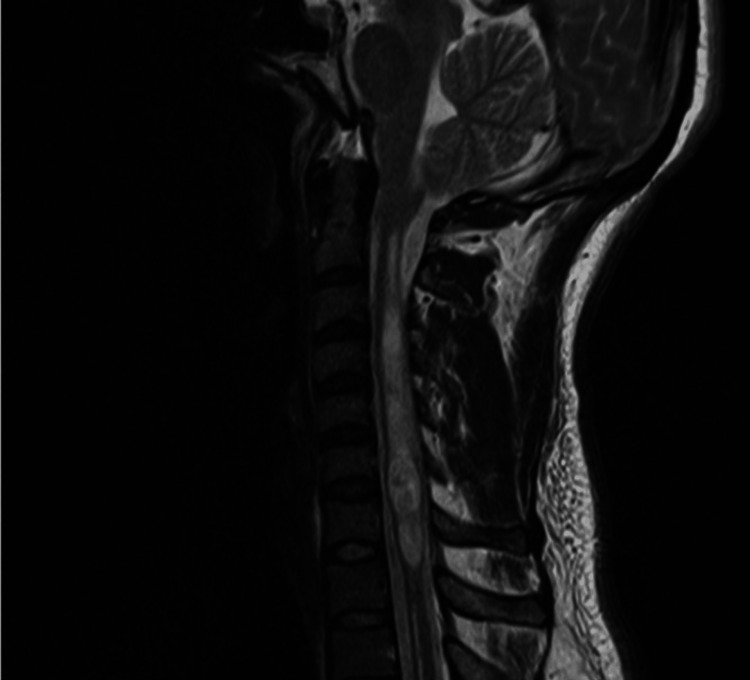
Representative preoperative MRI of a patient demonstrating cerebellar ectopia as well as associated spinal cord syrinx.
